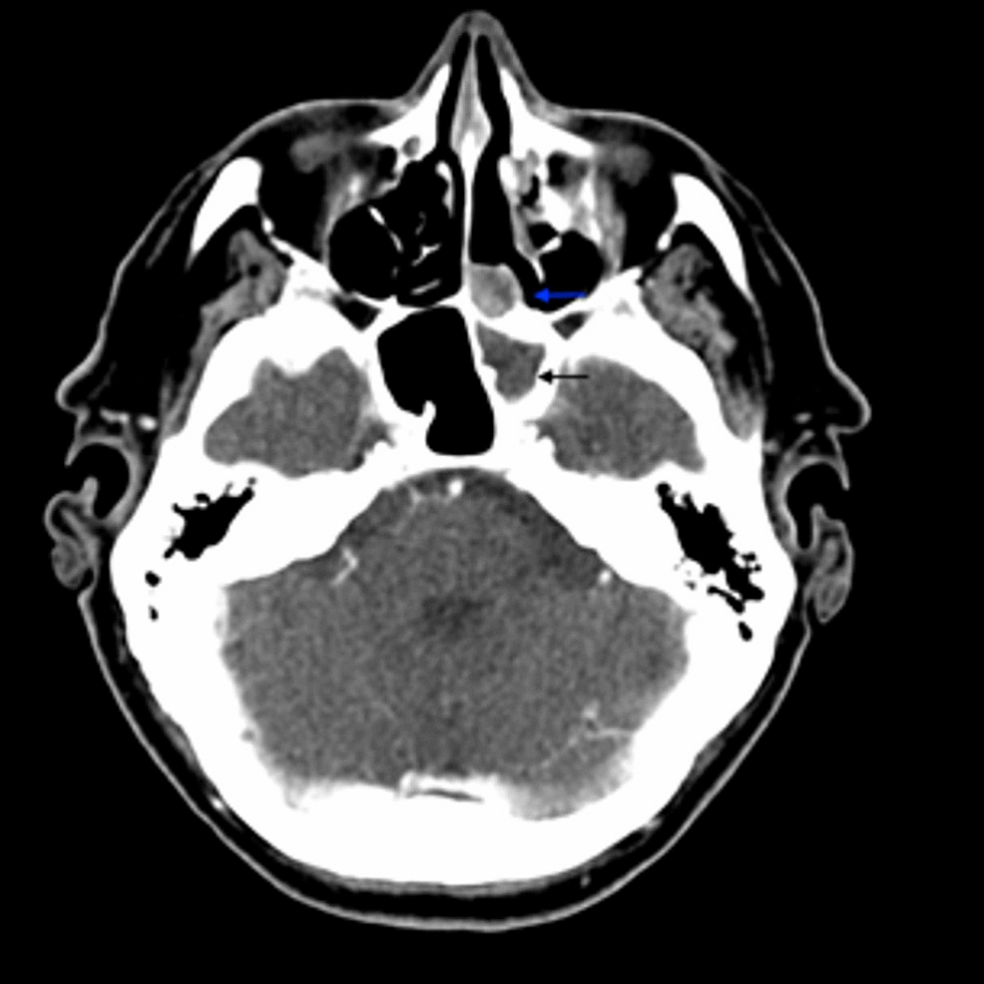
Axial view of contrast-enhanced CT showing a soft tissue density structure causing complete opacification of the left sphenoid sinus and extension into the adjacent ethmoid sinus(as demonstrated by black and blue arrows, respectively CT: computed tomography
